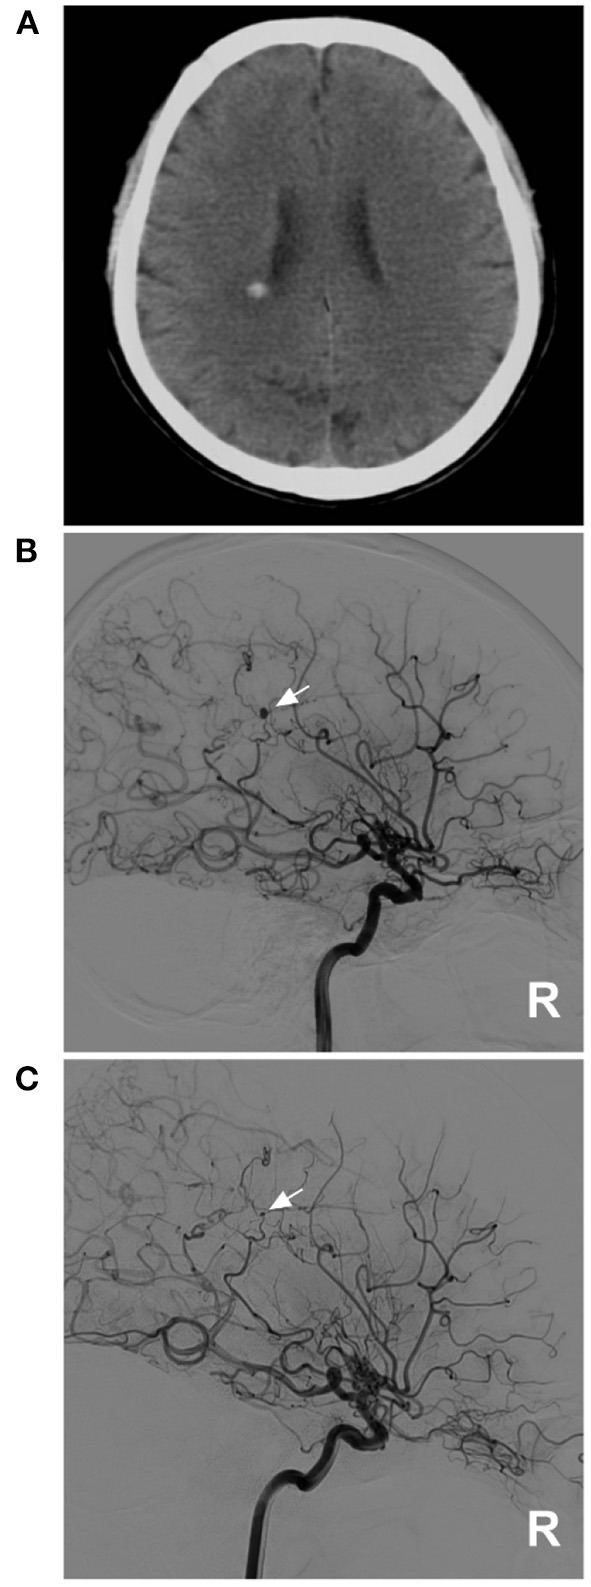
A diminishing aneurysm in case 1 in Table 2. (A) CT showed hemorrhage on the lateral ventricle wall. (B) DSA of the right ICA showed an aneurysm on the distal AchA (arrow), and MMD can be seen on this side. (C) One month later, repeat DSA of the right ICA showed that the aneurysm was diminishing, and only a remnant could be seen (arrow). AchA, anterior choroidal artery; CT, computed tomography; DSA, digital subtracted angiography; ICA, internal carotid artery; MMD, moyamoya disease; R, right.
